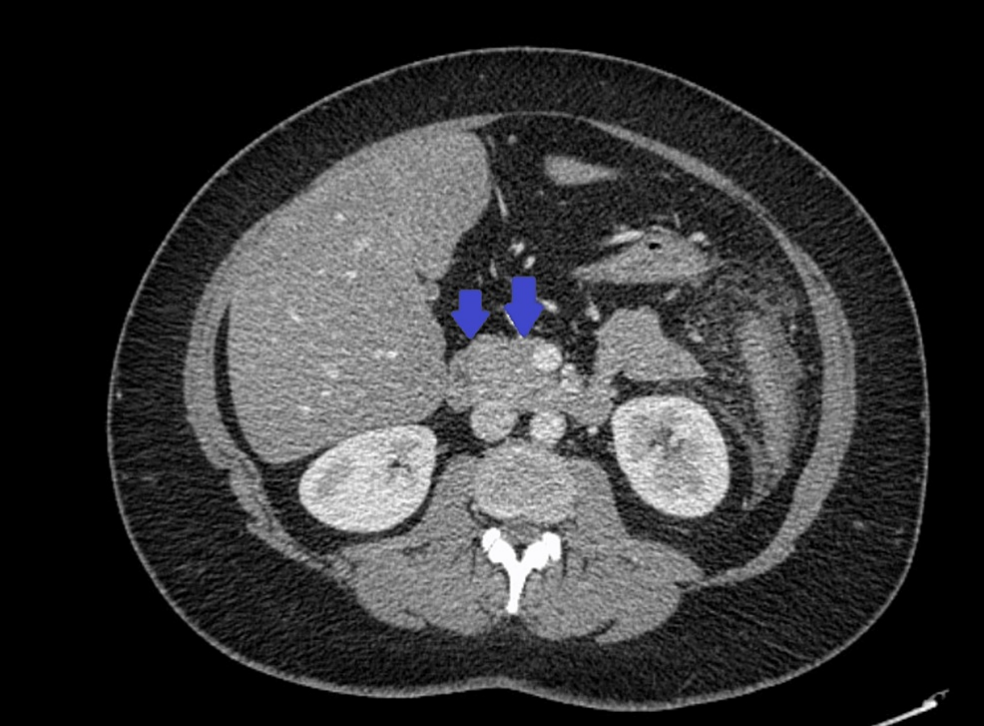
Computed tomography (CT) of the abdomen and pelvis with contrast showing acute pancreatitis (blue arrows)
